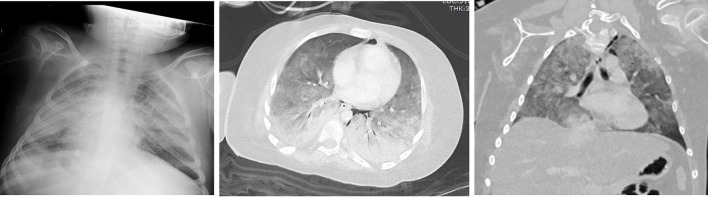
Male, 8 years old intubated. Chest X-ray and CT showed diffuse consolidation.
